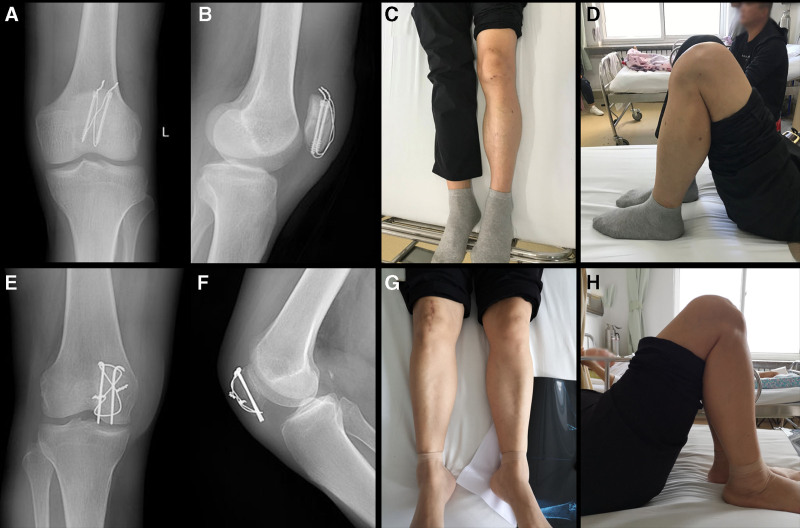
Clinical evaluation at 1-year follow-up. (A) Posteroanterior and (B) lateral X-rays of Patient 1 at 12 mo after surgery showing bone healing, (C) appearance of the minimally invasive surgical scar in the extension position, (D) evaluation of knee joint flexion. (E) Posteroanterior and (F) lateral X-rays of patient 2 at 12 mo after surgery showing bone healing, (G) appearance of the large surgical scar in the extension position, (H) evaluation of knee joint flexion.
